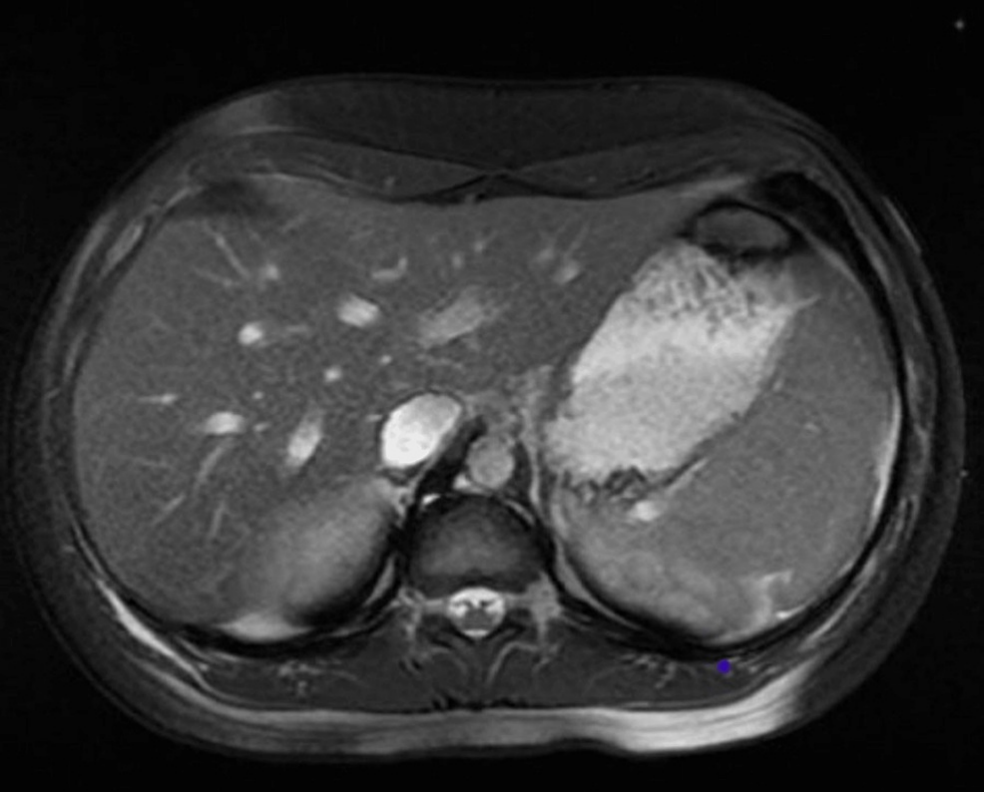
Magnetic resonance imaging (MRI) abdomen (axial) showing normal liver
